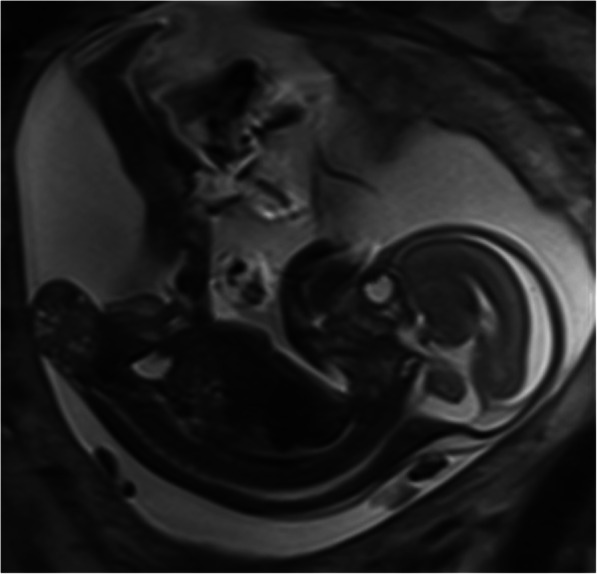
Magnetic Resonance Imaging (MRI) showing the sacrococcygeal teratoma, Type I and confirming the diagnosis
